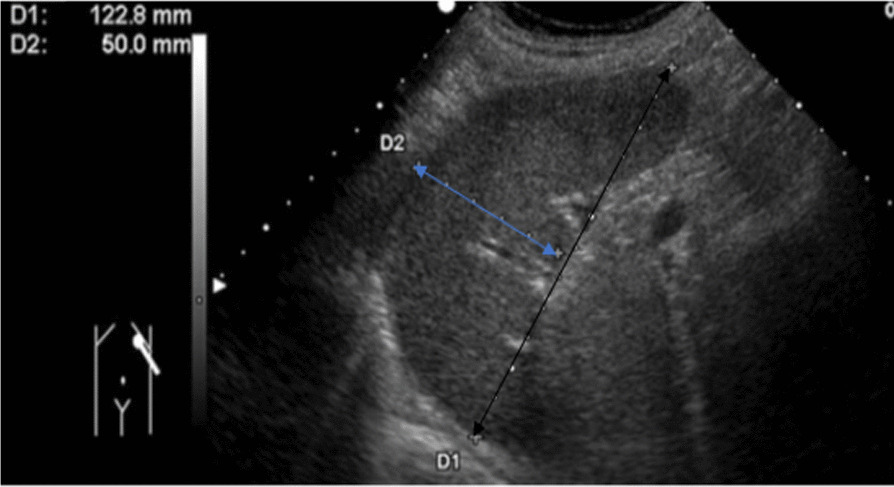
Spleen length (black arrow) and width (blue arrow) measured on longitudinal ultrasound scan
